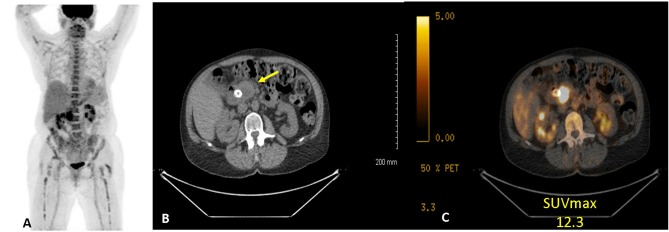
Example of ^18^FDG PET/CT of a women with PC. (A) anterior maximum intensity projection, (B) axial CT, and (C) axial fused CT. Study demonstrates a hypermetabolic (SUV_dx_ 12.3) primary pancreatic cancer.
